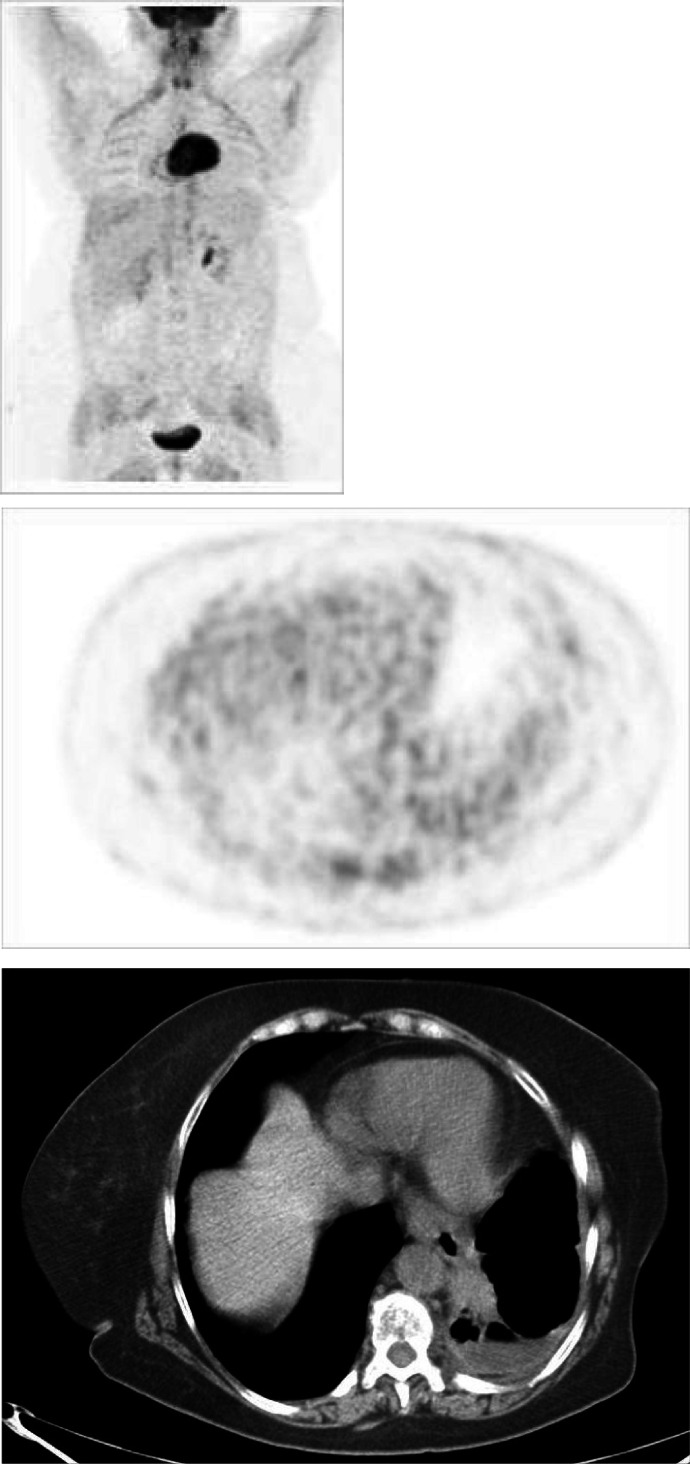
FDG PET (maximum intensity projection – MIP-image) showing no area of increased uptake. PET transaxial image shows no left lung increased uptake; corresponding transaxial CT image shows an equivocal subpleural area of consolidation in the left lower lobe. After lobectomy, this finding turned out to be benign (fibrosis and necrosis).
